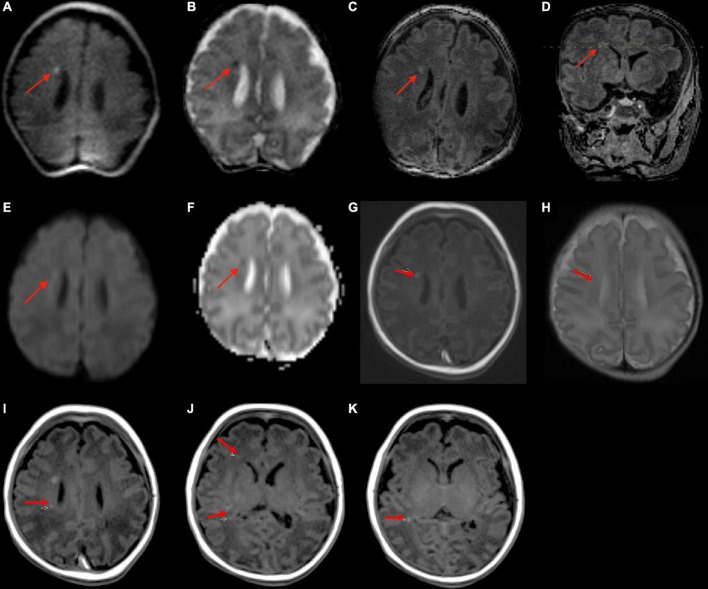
In a 5-day-old infant with a history of delivery complicated by shoulder dystocia requiring respiratory support and chest compressions, the point-of-care 1-Tesla DWI (A) and ADC (B) showed a punctate focus of reduced diffusion with associated T1 hyperintensity (C,D). Two days later, follow-up 3-Tesla MRI showed diffusion restriction (E,F), T1 hyperintensity (G), and T2 hypointensity (H) of the same lesion. There were four additional punctate foci of parenchymal injury with intrinsic T1 hyperintensity on 3-Tesla MRI (I–K), which could not be visualized on preceding day 1-Tesla scan. No transcranial ultrasound was obtained.
